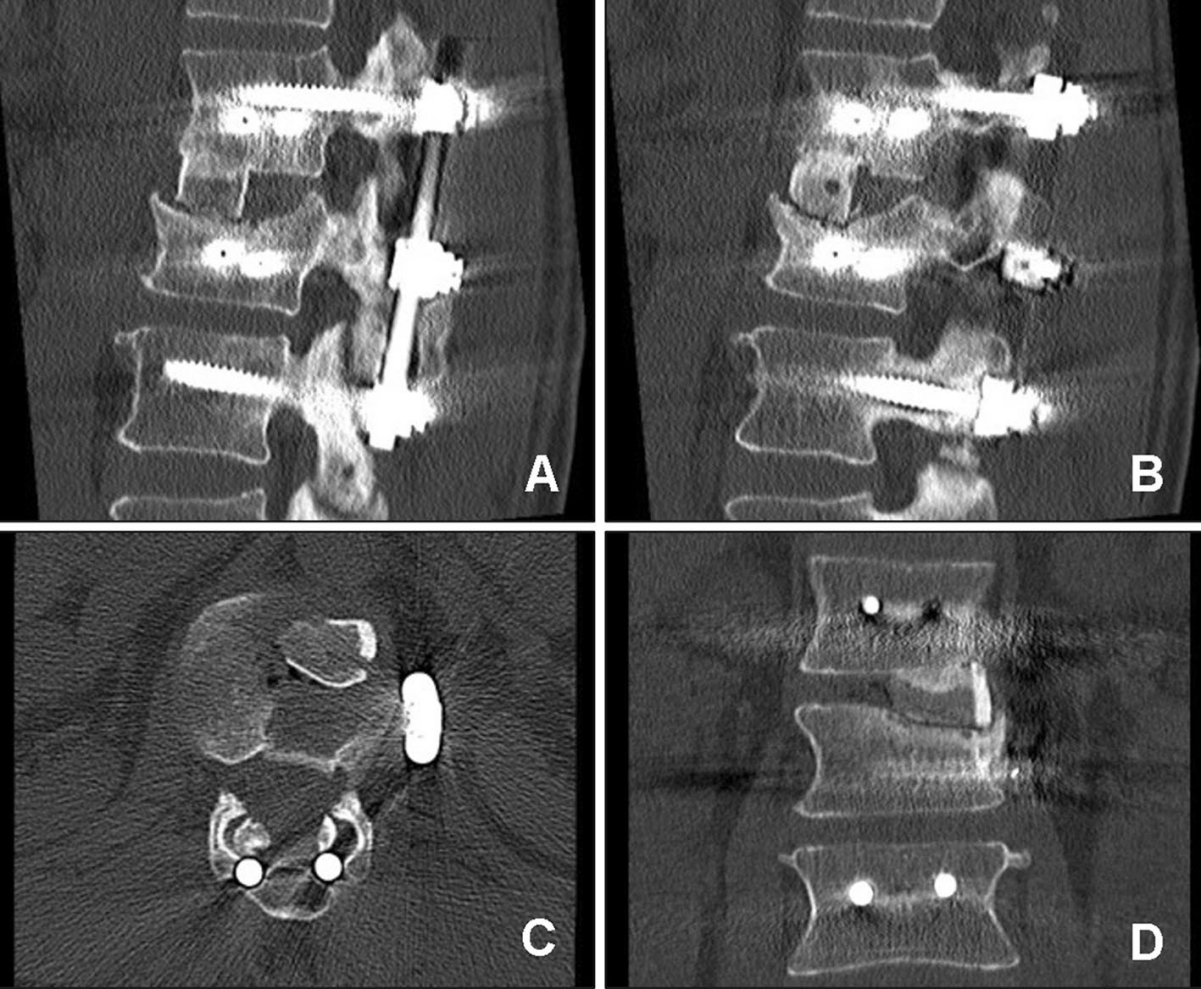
CT of a 41-year-old man (superior incomplete burst fracture of L2, Magerl et al.^19^ A3.1.1, AOSpine A3^1^) 11 months after short segment posterior instrumentation and anterior one-level spondylodesis using an ICBG, showing unipolar nonfusion between the lower part of the iliac crest bone graft and the adjacent vertebrae (L3).
